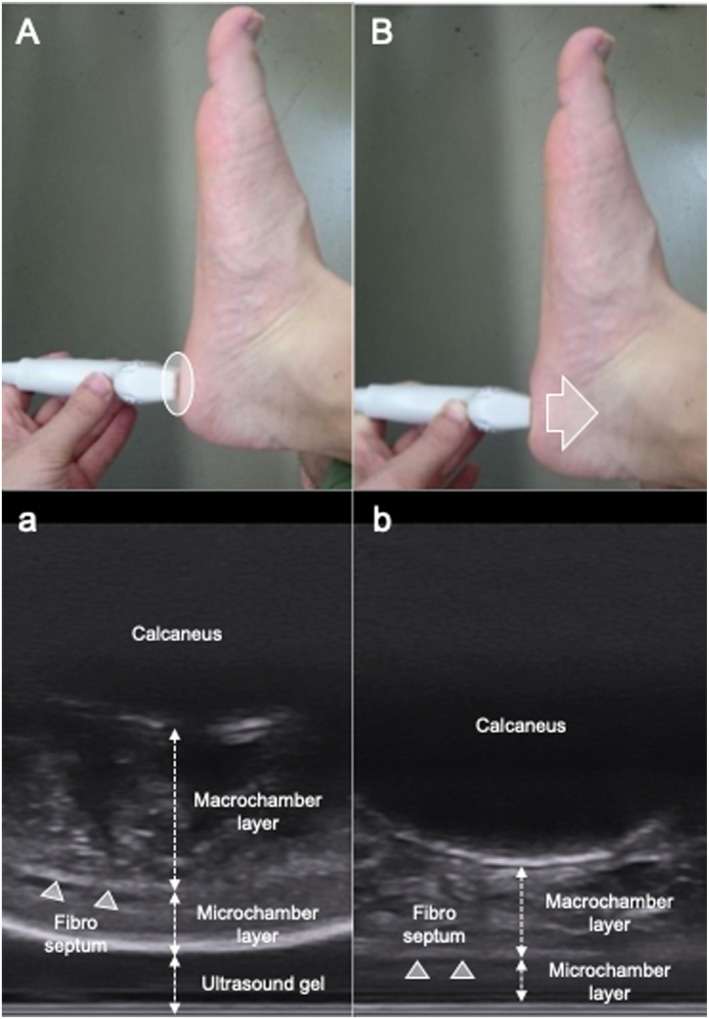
Method for assessing heel fat pad thickness using an ultrasound. (A) This is an image taken when measuring thickness without compression. Ultrasound gel was placed between the heel fat pad and the ultrasound probe to prevent the ultrasound probe from directly touching the skin and applying pressure. (a) Is an actual ultrasound image of the heel fat pad without compression. (B) This is an image taken when measuring thickness with compression. Maximum pressure was applied. (b) Is an ultrasound image of the heel fat pad with actual compression.
